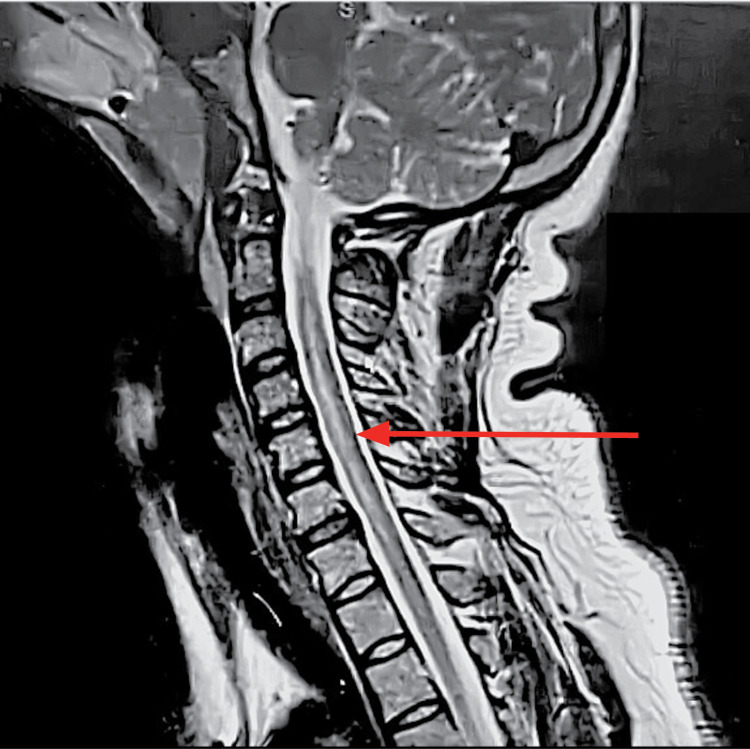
Sagittal, T2-weighted MRI of the cervical spine Red arrow indicating a hyperintense lesion along the central grey matter extending from lower medulla-T1
